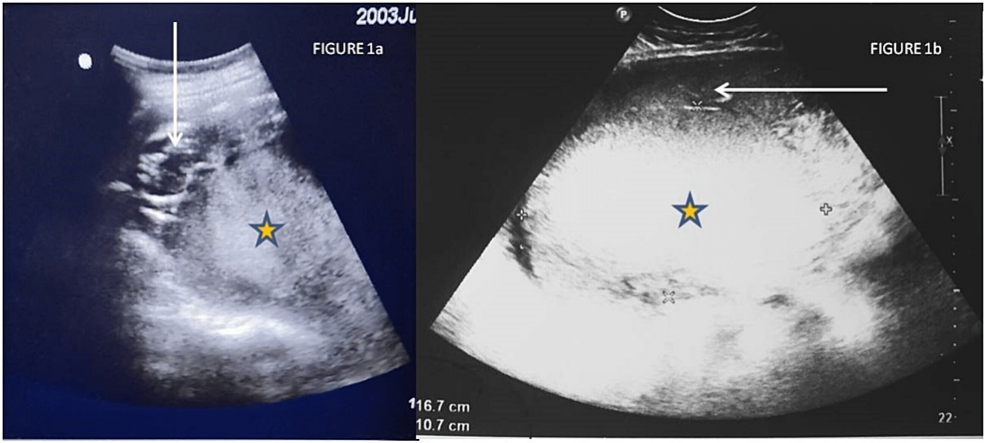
1a: Transabdominal ultrasound showing the placenta (arrow) pushed anteriorly by the mass (star). 1b: Transabdominal ultrasound showing the intrauterine homogeneous mass measuring 16.7 × 10.7 cm (star) in relation to the placenta (arrow).
